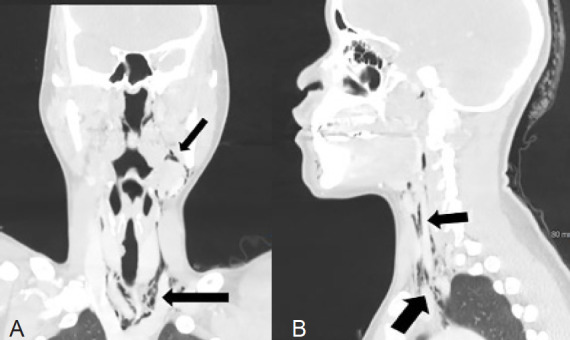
(A) Computed tomography (CT) with intravenous contrast (coronal) demonstrating extension of the subcutaneous emphysema from left lower molar tooth, along both the anterior and posterior triangles extending up to the retrosternal area and into the mediastinum (arrows). (B) CT with intravenous contrast (sagittal) demonstrating extension of the subcutaneous emphysema from the left lower molar tooth into the retropharyngeal space and mediastinum (arrows).
